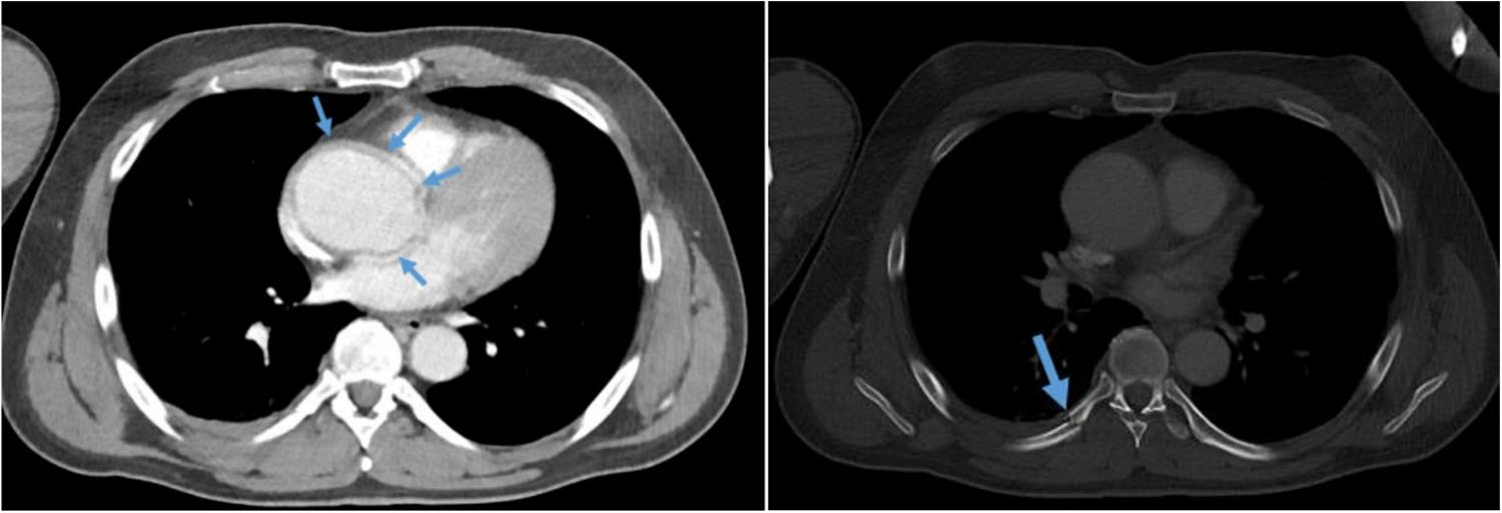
Polytrauma CT after the accident. Left, axial reconstruction in soft tissue window with sickle-shaped contrast signal surrounding the ascending aorta. Right, axial reconstruction in bone window with paravertebral rib fracture of the right 7^th^ rib
